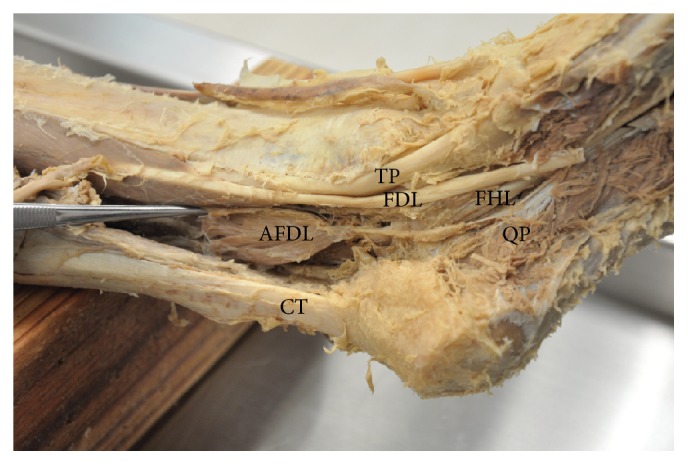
Dissection of left leg and foot to show accessory flexor digitorum longus muscle (AFDL: accessory flexor digitorum longus muscle; CT: calcaneal tendon; FDL: flexor digitorum longus tendon; FHL: flexor hallucis longus tendon; TP: tibialis posterior tendon; QP: quadratus plantae muscle).
